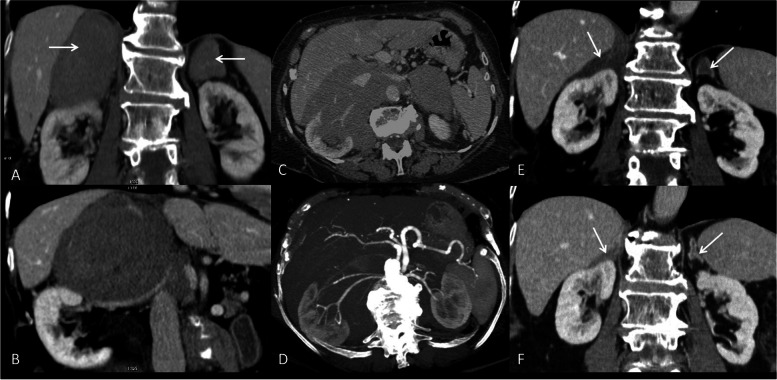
First CT scan showing A asymmetric enlargement of the right adrenal mass imprinting the liver and displacing caudally the normal gland; B detail of the right adrenal mass that displaces the surrounding structures. The kidney is displaced caudally and renal vessels are marked but without signs of infiltration; C A second CT scan revealing the dimensional increase of the adrenal masses. The right one incorporates all the surrounding vascular structures up to the left vascular bundle; D Maximum Intesity Projection (MIP) reconstruction shows that the right vessels near the adrenal mass are stretched but without signs of infiltration; E Three months after therapy CT scan revealed a complete remission of the right mass. The adrenal gland shows normal morphology with densification of the surrounding fatty tissue. The left adrenal mass is greatly reduced in size; F Eighteen months after diagnosis CT scan showed a complete remission of both the adrenal masses
